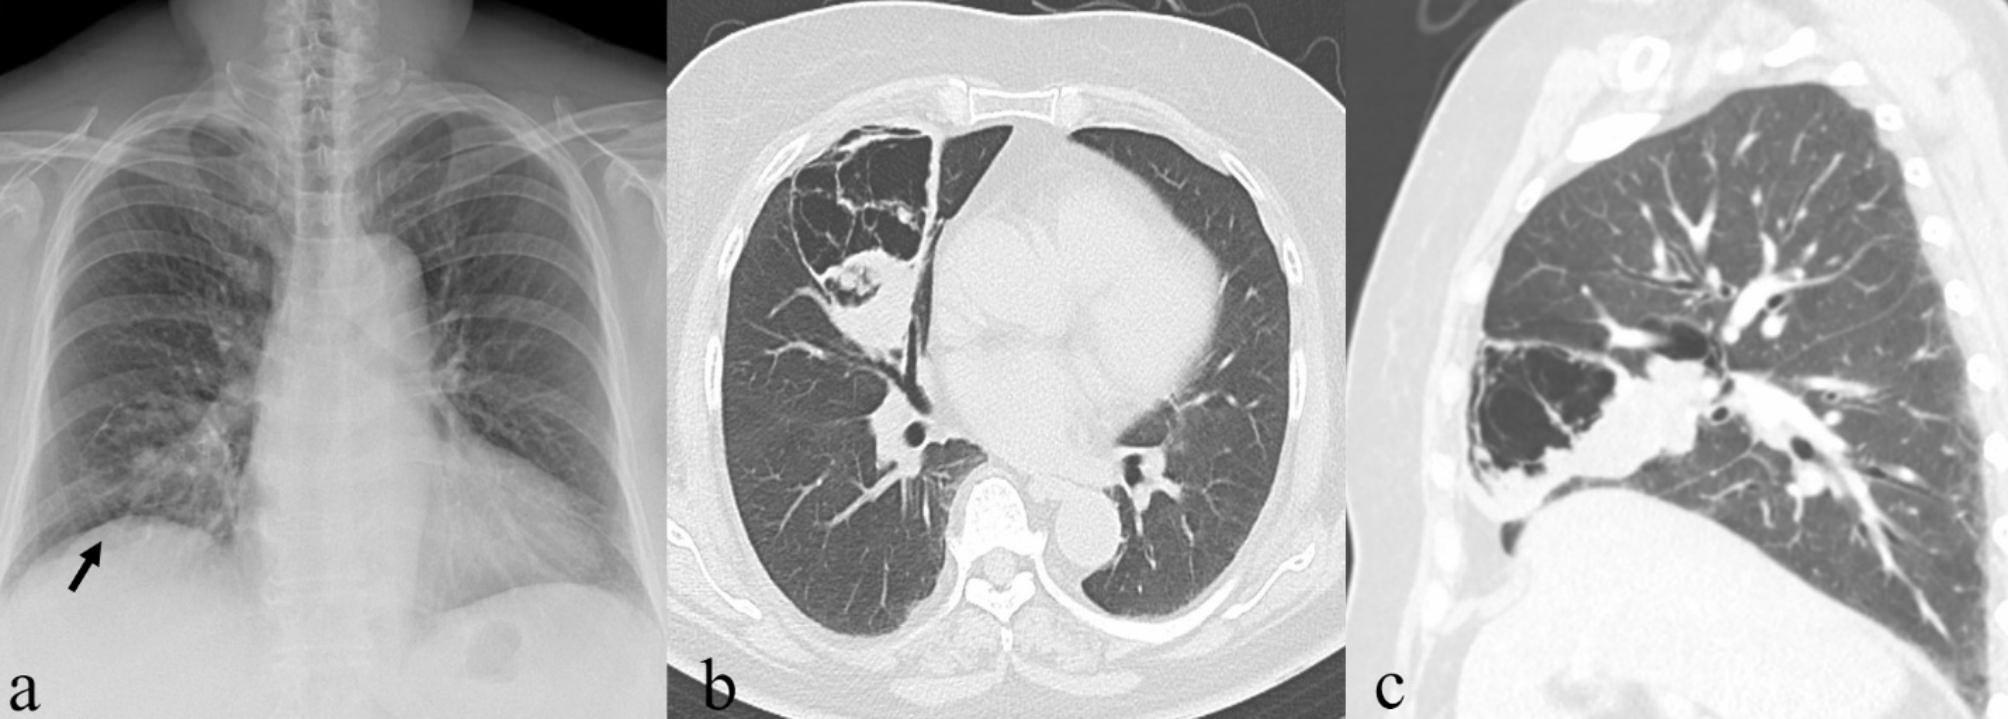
Preoperative imaging studies: (A) cystic area (black arrow) and ground-glass opacity of right hilar region detected by chext X-ray; and (B, C) computed tomography of chest (axial and sagittal views) demonstrating large cystic area (8.8 × 6.1 cm) and solid growth (4.9 cm) of right middle lobe
